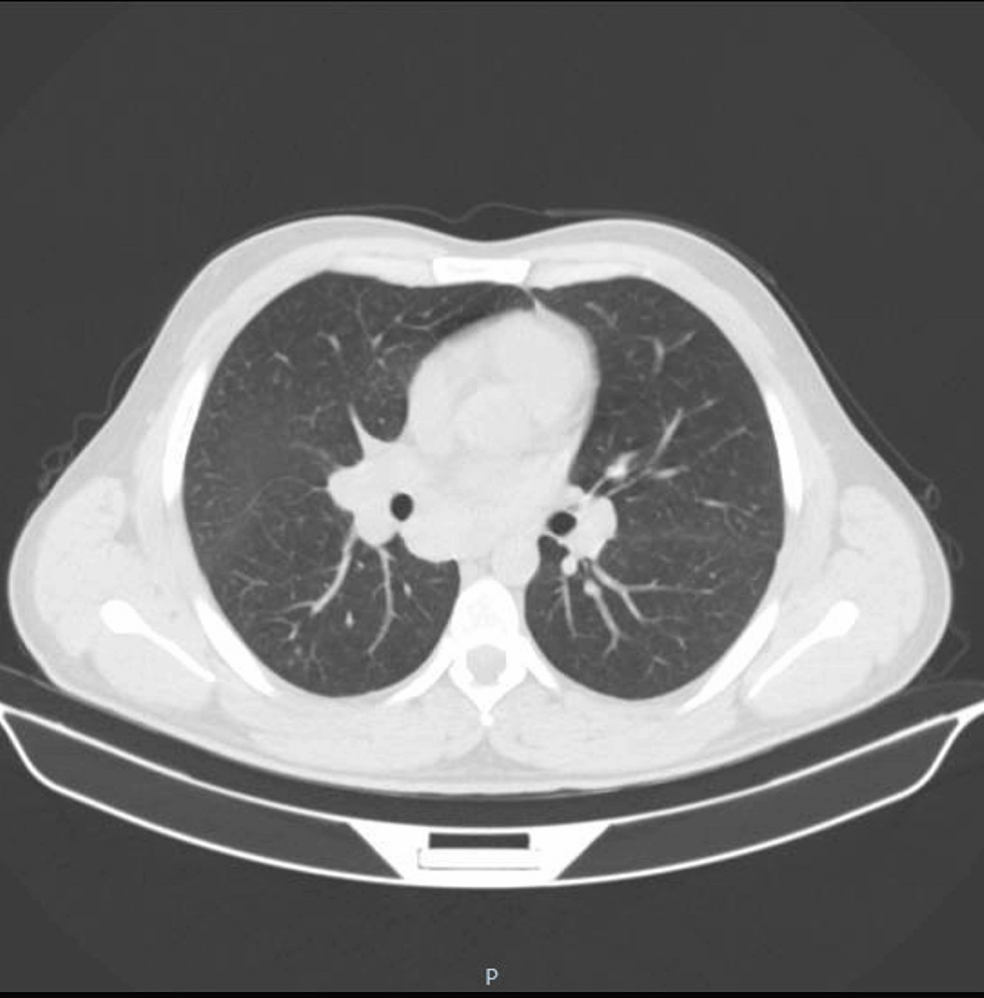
CT scan showing perihilar lymphadenopathy
